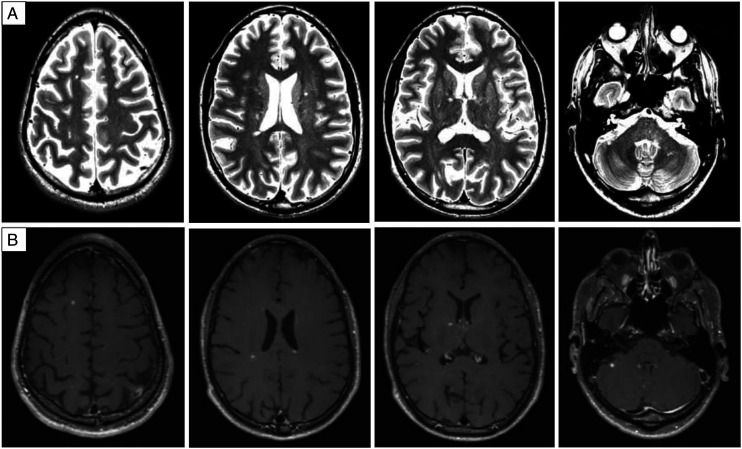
Brain MRI before start of ocrelizumab. April 24, 2020. Brain MRI after multiple sclerosis relapse and high-dose intravenous methylprednisolone therapy, while on treatment with dimethylfumarate, revealed multiple T2 hyperintense white matter lesions, partially in typical areas for multiple sclerosis (A: T2-weighted images) and several of them with contrast enhancement (B: T1-weigted images with gadolinium). While this figure shows only some representative slices, the examination demonstrated 15 new lesions compared to the previous MRI nine months earlier.
